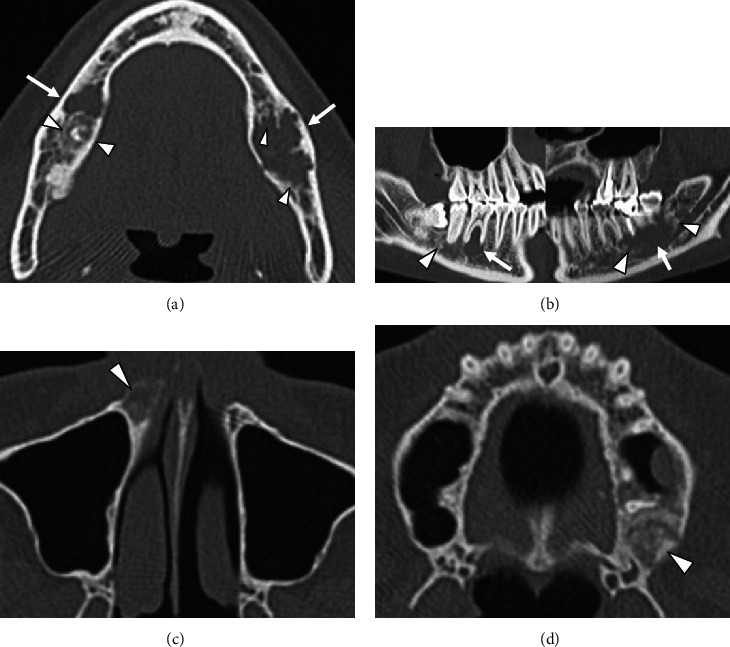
CT images. (a, b) Well-defined, low-density lesions (*arrows*) with scalloped border, which accompanies a well-defined high-density appearance (*arrowheads*) are observed in the right mandibular molars on transverse and sagittal images. The left lesion shows mixed main low- (*arrows*) and partial high- (*arrowheads*) density areas with an unusual, marked irregular border in the molar region. The right lesion shows a unilocular, slightly irregular border. (c, d) Transverse CT images show high-density lesions in the right anterior and left posterior maxilla (*arrowheads*). CT: computed tomography.
